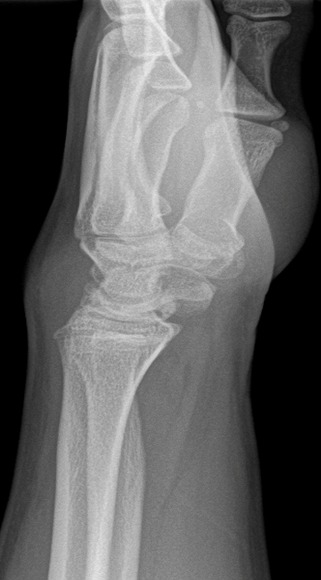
Lateral radiographs wrist showed a densification of the lunate with a flattened and irregular appearance of the lunate, without signs of osteoarthritis
